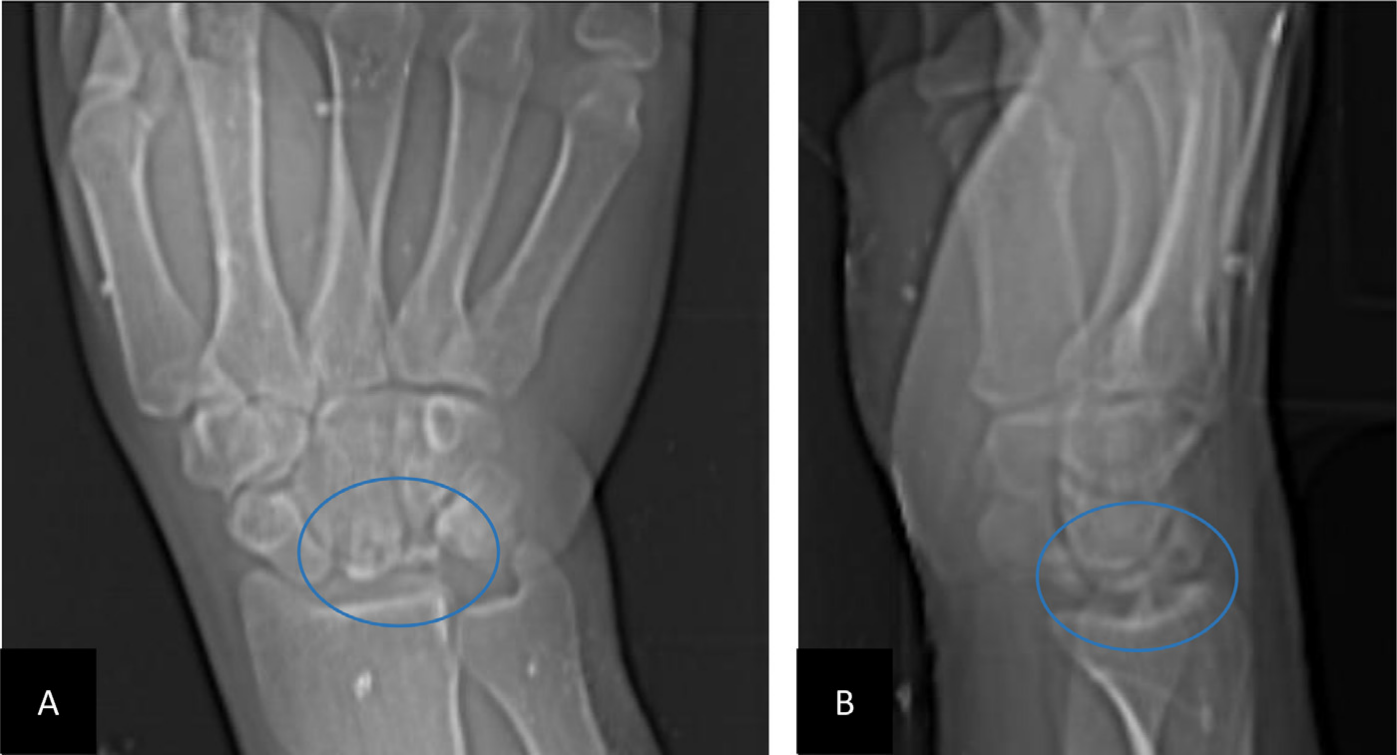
X-ray of the right wrist: the anteroposterior (A) and lateral (B) views revealed a collapsed and heterogeneous lunate with no involvement of the other carpal bones.
